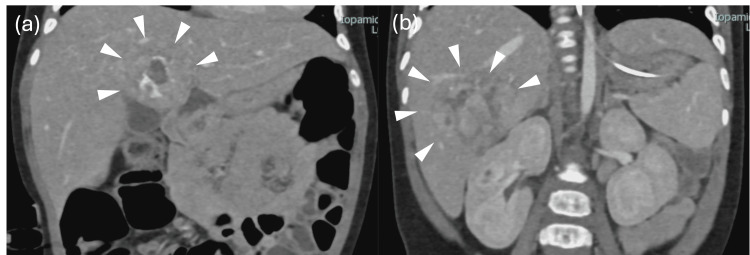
Contrast-enhanced computed tomography (CT) findings (a) The segment 4 tumor and (b) the segment 6 tumor. The white arrowheads indicate the tumor.
